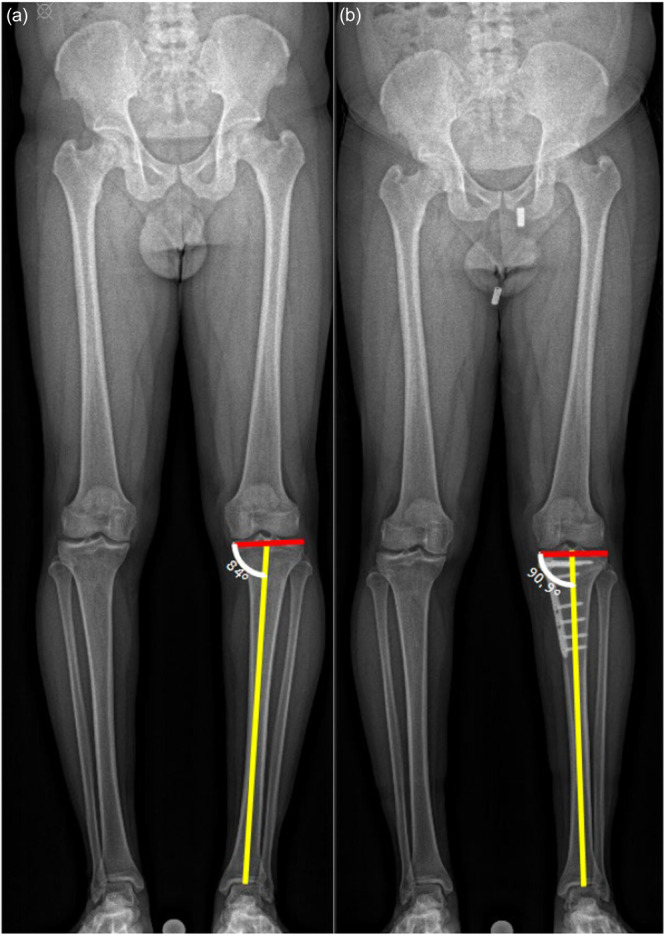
Weight‐bearing long leg radiograph with the coronal alignment of the right leg for the measurement of the MPTA. Weight‐bearing long leg radiograph (LLR) with the measurement of the mechanical proximal tibial angle (MPTA) of the right leg preoperatively (a) and postoperatively (b). The red line determines the tangent to the tibial plateau and the yellow line the mechanical axis of the tibia.
